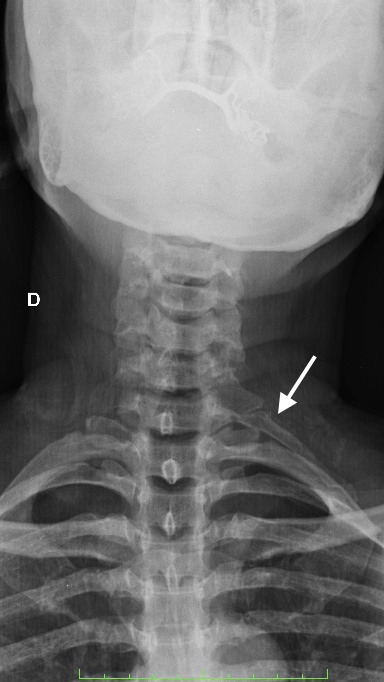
Anteroposterior cervical X-ray from the second case showing a cervical rib joined to the first right rib (white arrow).
